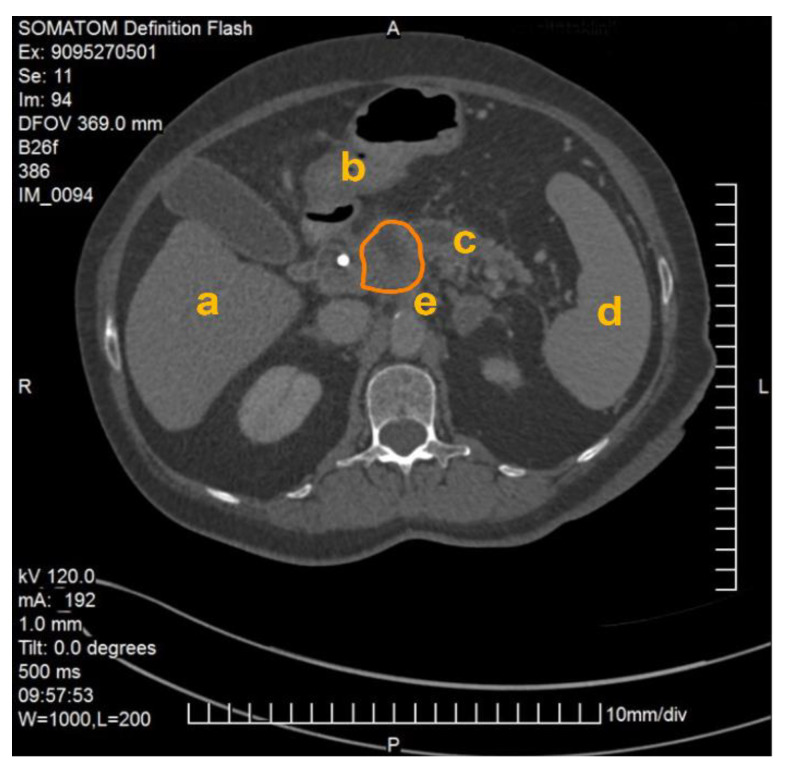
CT scan of a patient with locally advanced pancreatic ductal adenocarcinoma. Visible are the pancreas with a splint in the head of the pancreas as well as the liver (a), the gastroduodenal junction (b), the dilated pancreatic duct (c), the spleen (d), the abdominal aorta and the origin of the coeliac trunc (e). The PDAC tumor is outlined in orange.
